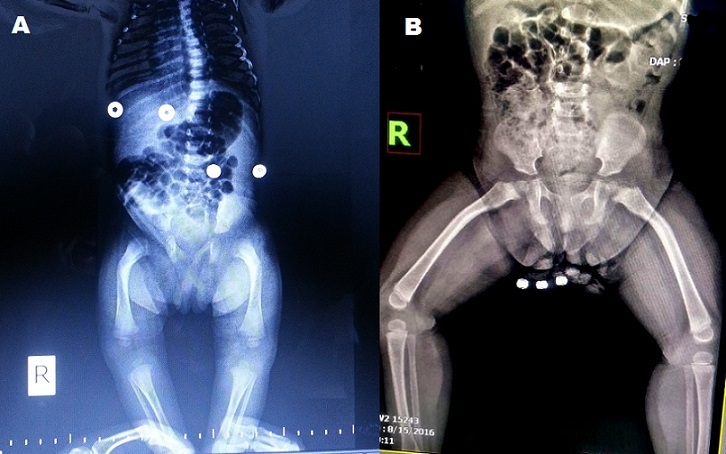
Postnatal radiography shows mildly curved femurs and osteopenia: (A) First day after birth, (B) One year later after birth
